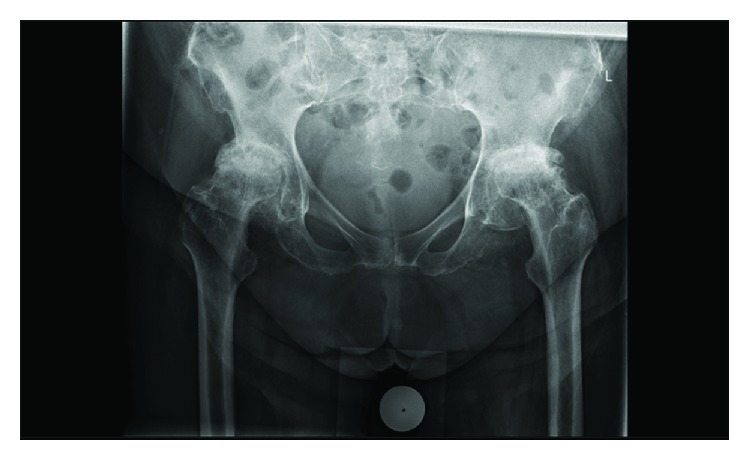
Preoperative anteroposterior X-ray of the pelvis.
